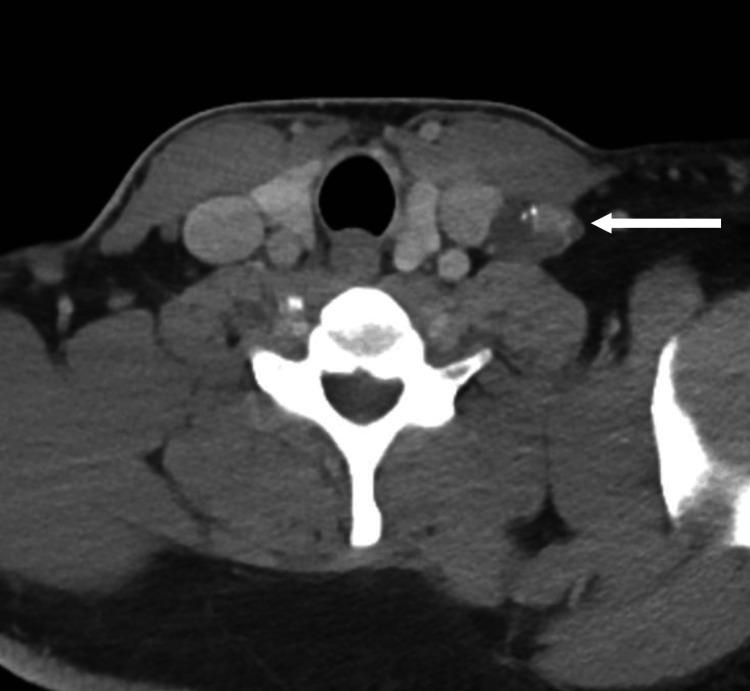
Neck imaging. CT scan showing left middle pole thyroid nodule (arrow) as well as a possible lymph node with calcifications.
